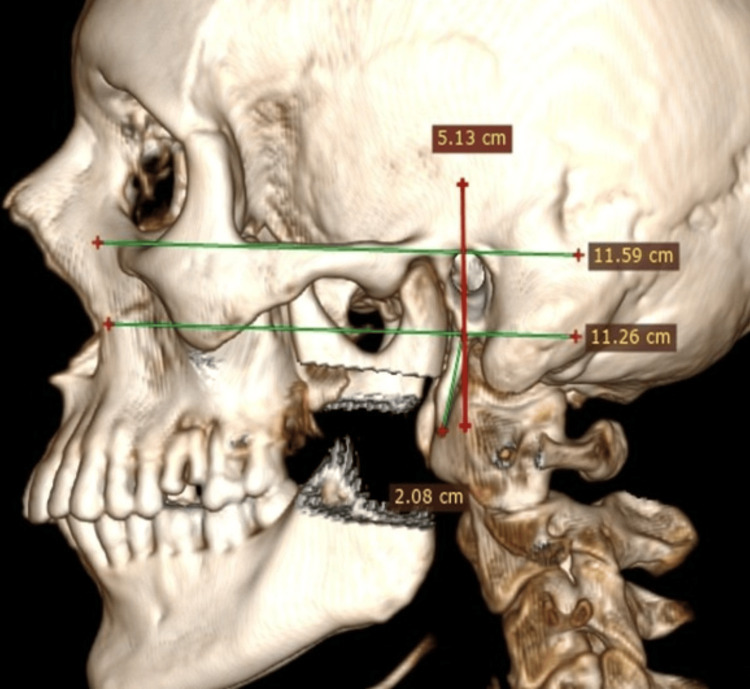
Normal length (<3 cm long) of styloid process on lateral view on 3D CT.
